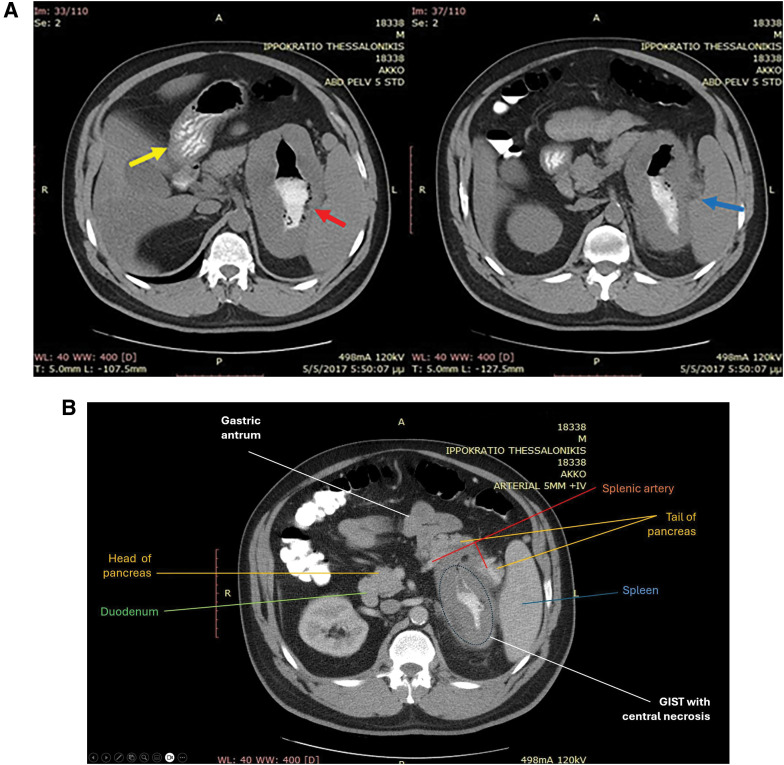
(A) Abdominal computed tomography scan of the patient. Two diverging lumens are filled with gastrografin: 1 lumen shows visible gastric folds (yellow arrow), while the other contains undigested material (red arrow). The mass appears to be adhered to the tail of the pancreas and the splenic hilum (blue arrow). (B) Anatomical relationships of the tumor with the surrounding structures, as seen on the abdominal computed tomography scan. Possible infiltration or strangulation of the tail of the pancreas. A “blurring” is described between the tumor and the splenic artery. The tumor also appears to be adhered to the splenic hilum. GIST, gastrointestinal stromal tumor
